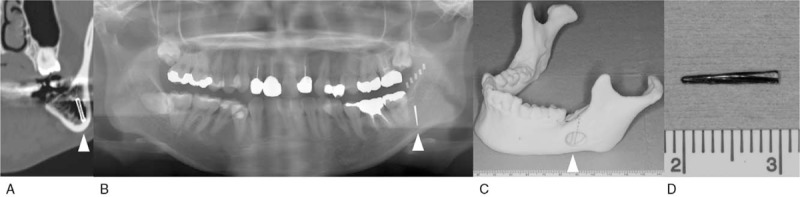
Case 1. (A) Preoperative computed tomography. (B) Preoperative panoramic radiograph with a stent that enclosed the radiopaque materials. (C) Three-dimensional model created based on the preoperative computed tomography. (D) The foreign body.
